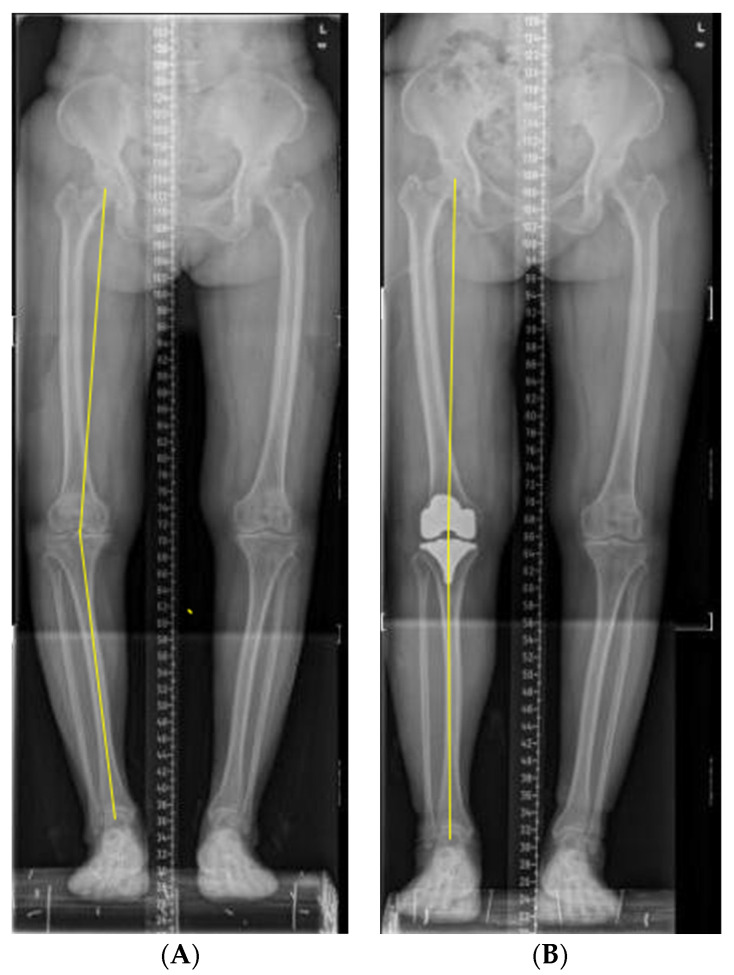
The static lower limb alignment was measured on the radiograph. (A) Before operation; (B) after operation.
